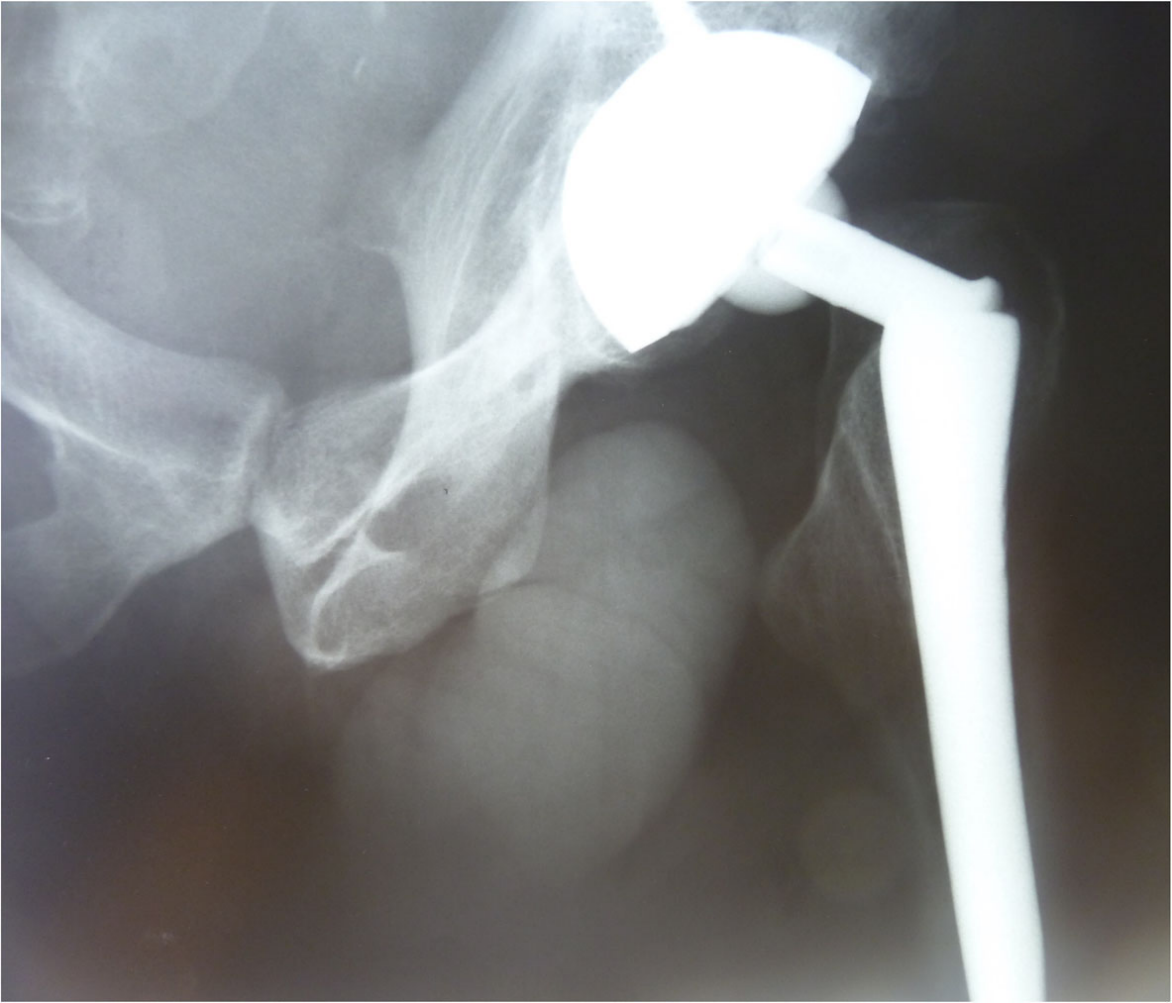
Emergency room X-ray of the left hip with fractured modular femoral neck 3 years after primary THA
